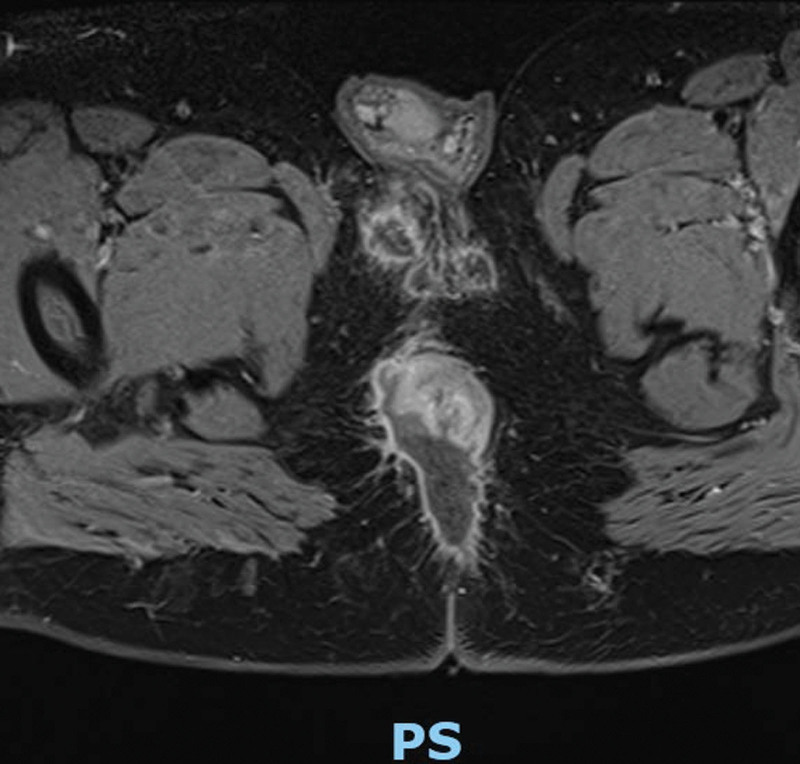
MRI showed anal sphincteric damage due to severe anorectal sepsis and multiple surgical drainage. MRI = magnetic resonance imaging.
